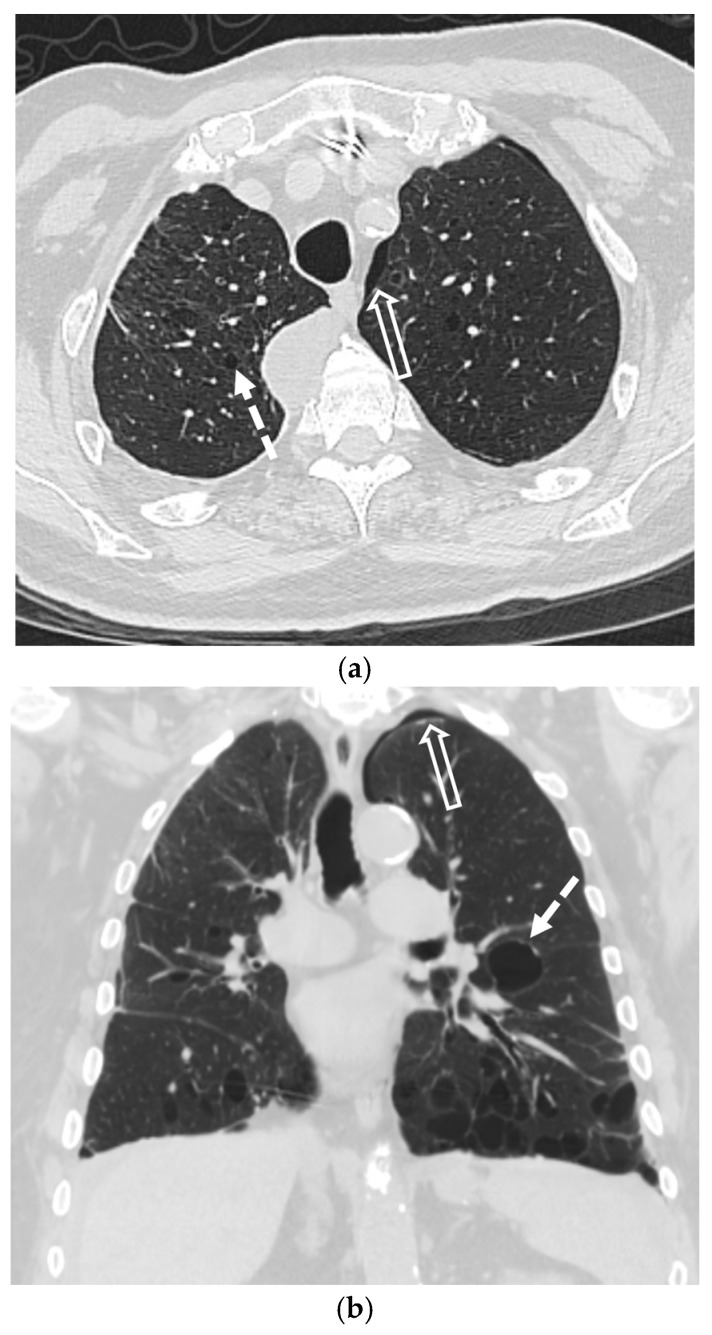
81-year-old male with Birt–Hogg–Dubé syndrome presents with left-sided chest pain. Axial and coronal CT images in lung window (a,b) demonstrate multiple bilateral thin-walled pulmonary cysts (dashed white arrows) of varying size with basilar predominance, along with trace left apical pneumothorax (open white arrow).
